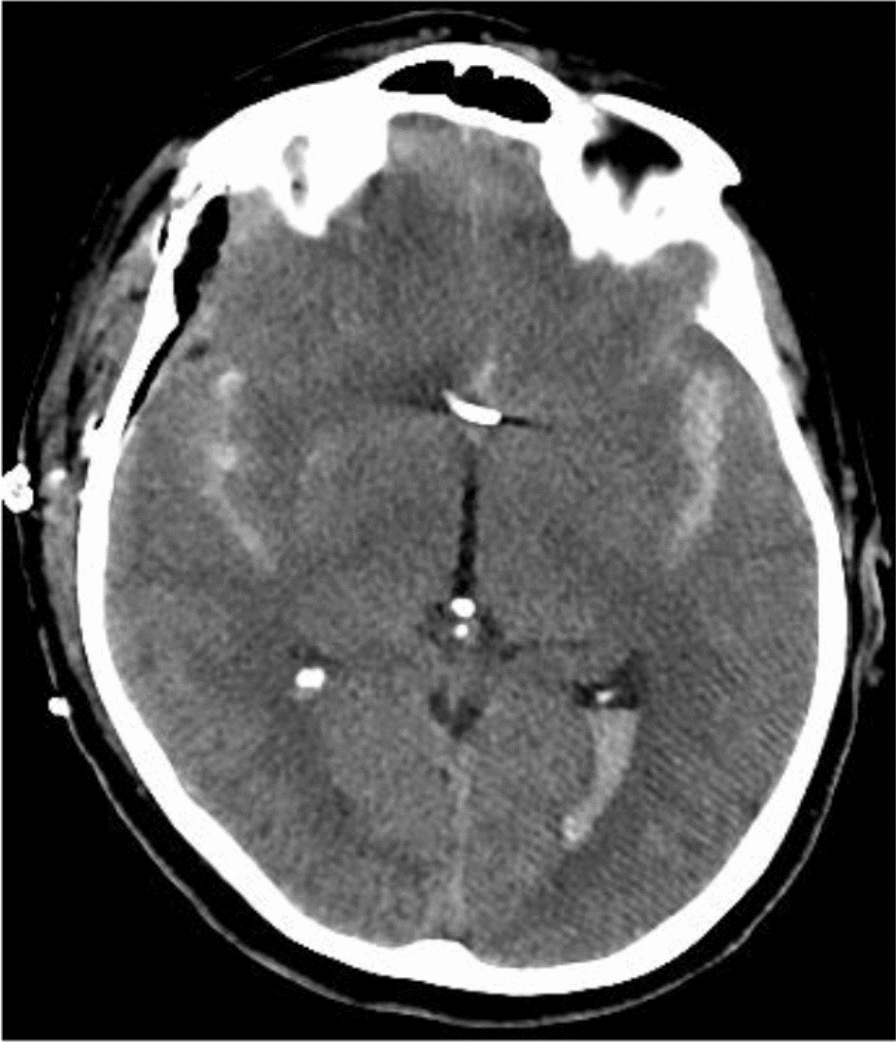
Computed tomography scan showing subarachnoid hemorrhage and the clip on the aneurysm
